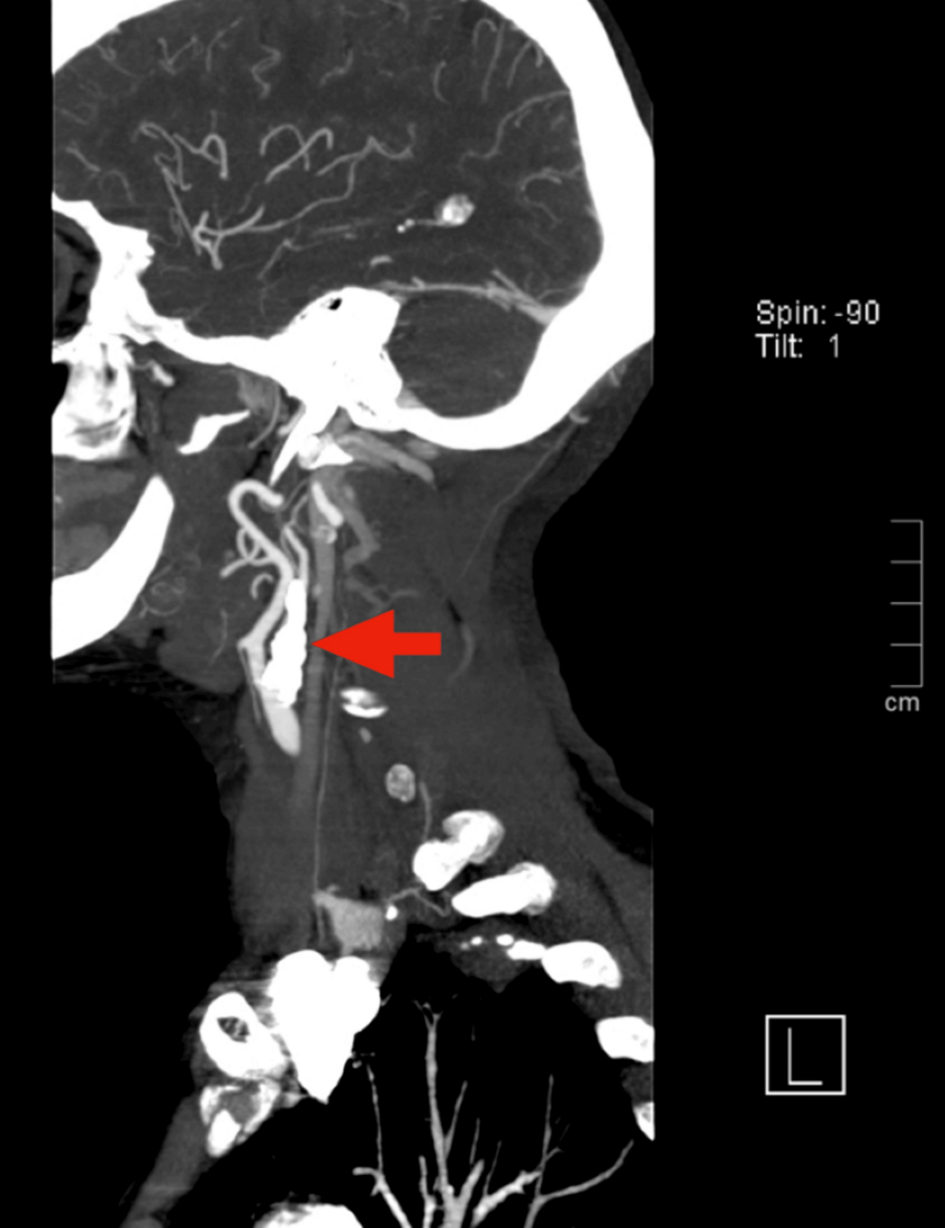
Sagital cervicoencephalic CT angiography shows calcified atherosclerotic plaques in the right carotid artery
